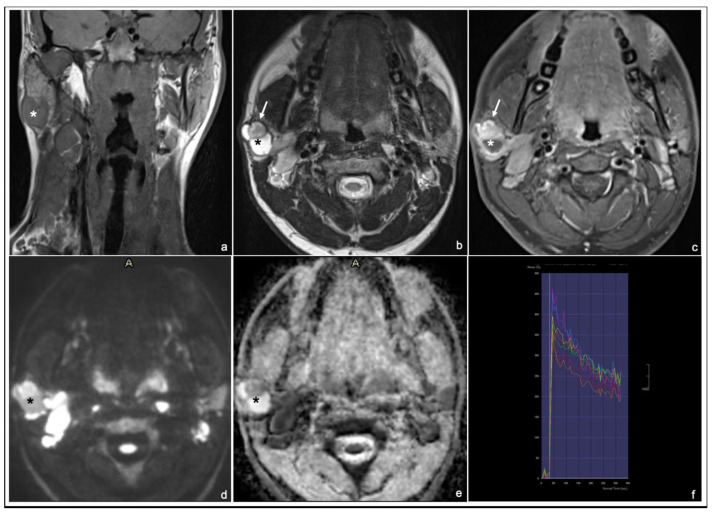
15 years old boy with low-grade mucoepidermoid Ca of the right parotid gland: coronal T1 TSE (a), axial T2 TSE (b), late T1 TSE FS + Gd (c), DWI and ADC map (d,e) and DCE signal intensity-time S(t)-curve type B (f). * tumor. The solid portion of the lesion (arrows) shows an intense enhancement on late T1 postcontrast sequence (normalized late T1 + Gd SI ≥ 2) (c) and appears hypointense in T2 (normalized T2 SI < 3.5) (b).
